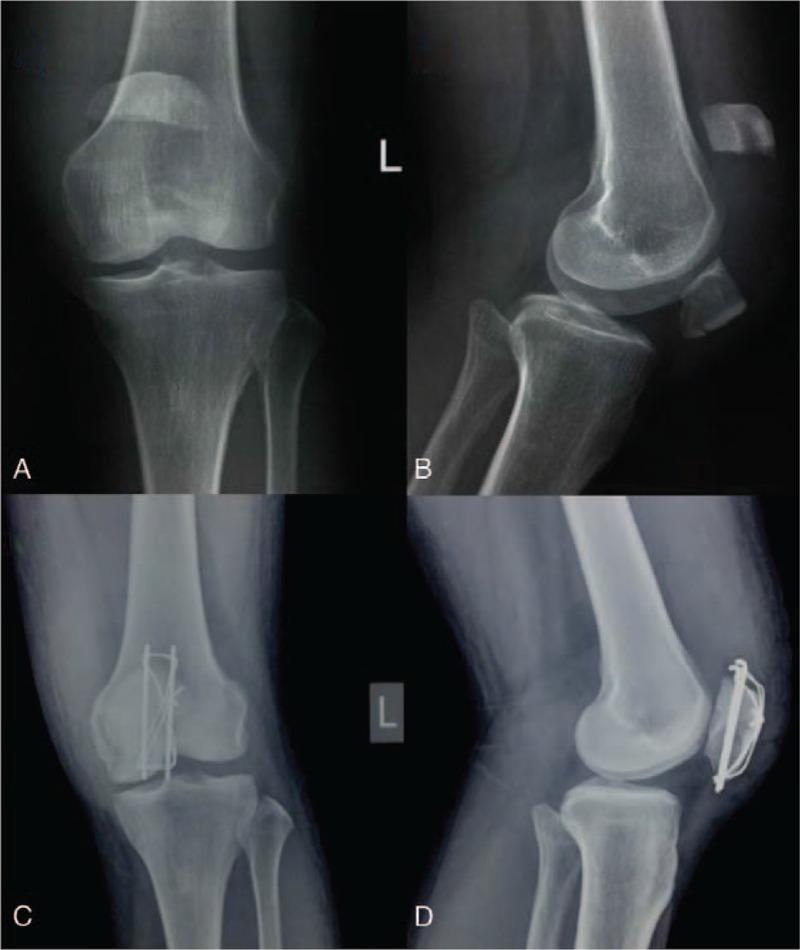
(A) Preoperative anteroposterior and (B) lateral radiographs showing a transverse fracture of the left patella with an inferior pole occult fracture. (C) Postoperative anteroposterior and (D) lateral radiographs showing an anatomic reduction and rigid fixation.
